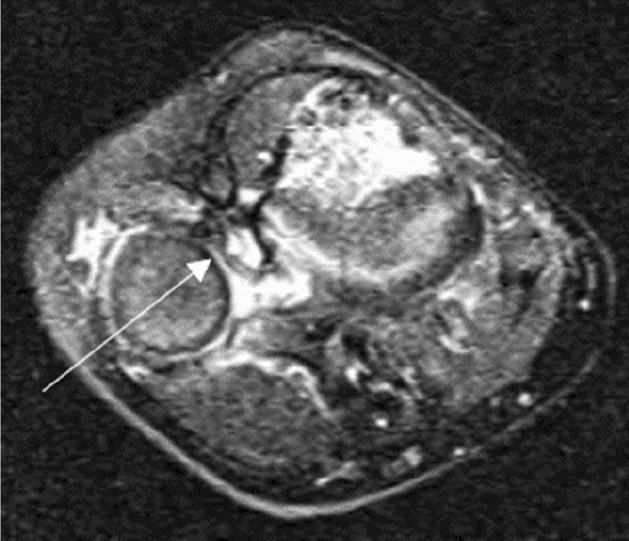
T2-weighted images displayed obvious tear of the annular ligament (the arrow) of the case 14.
